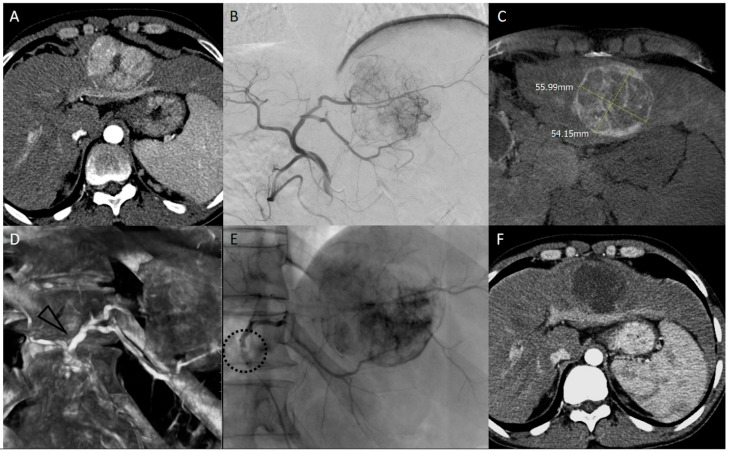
Clinical case of DEM-bTACE in a 41-year-old man with HBV-related cirrhosis, performed as bridging to orthotopic liver transplant. Pre-procedural arterial phase CT (A) showed a single 5.5 cm hepatocellular carcinoma in segment II-III. The transfemoral digital subtraction angiography of the common hepatic artery (right oblique 25°) (B) and the Dual-Phase Cone Beam CT ((C), delayed phase) confirmed the HCC nodule and vascular anatomy. VR reconstruction obtained from the arterial phase Cone Beam CT dataset (D) depicts the correct segment of the artery for microballoon positioning. After balloon inflation (dotted circle), embolization (E) was performed with 100 and 200 μm microspheres loaded with 100 mg of epirubicin. CT performed at 1 month ((F), arterial phase) showed a complete response. The patient underwent an orthotopic liver transplant 7 months after bTACE.
